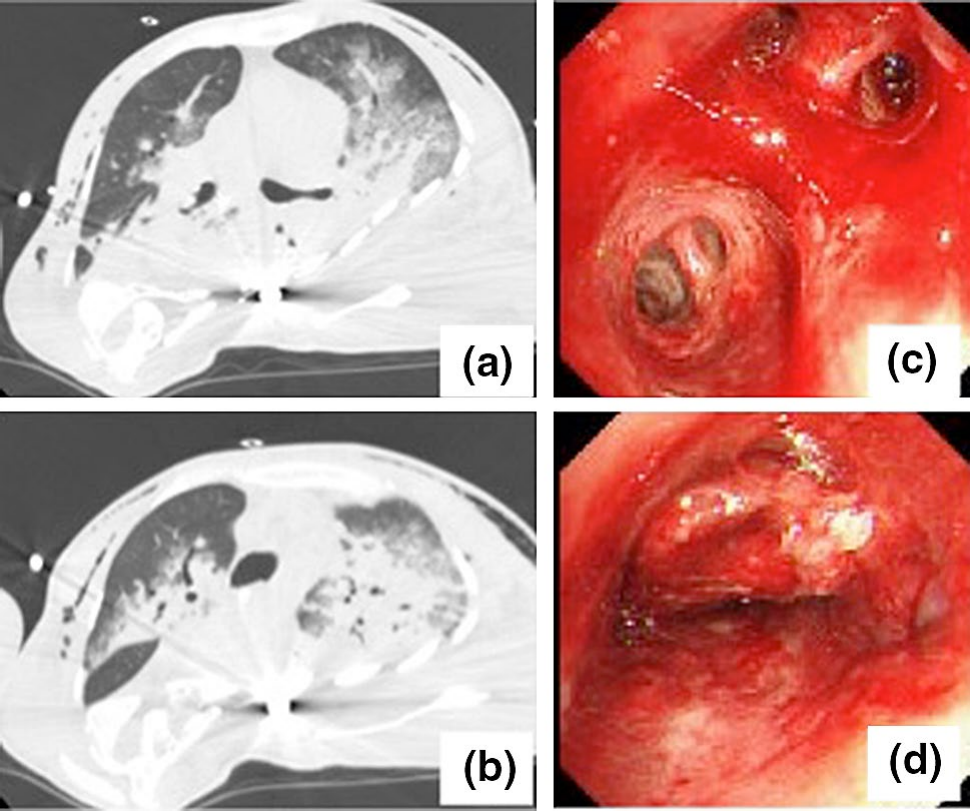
Case 2: a, b CT scan on arrival. c, d Bronchoscopy after hemoptysis shows bleeding and blood clot occluding the left main bronchus
